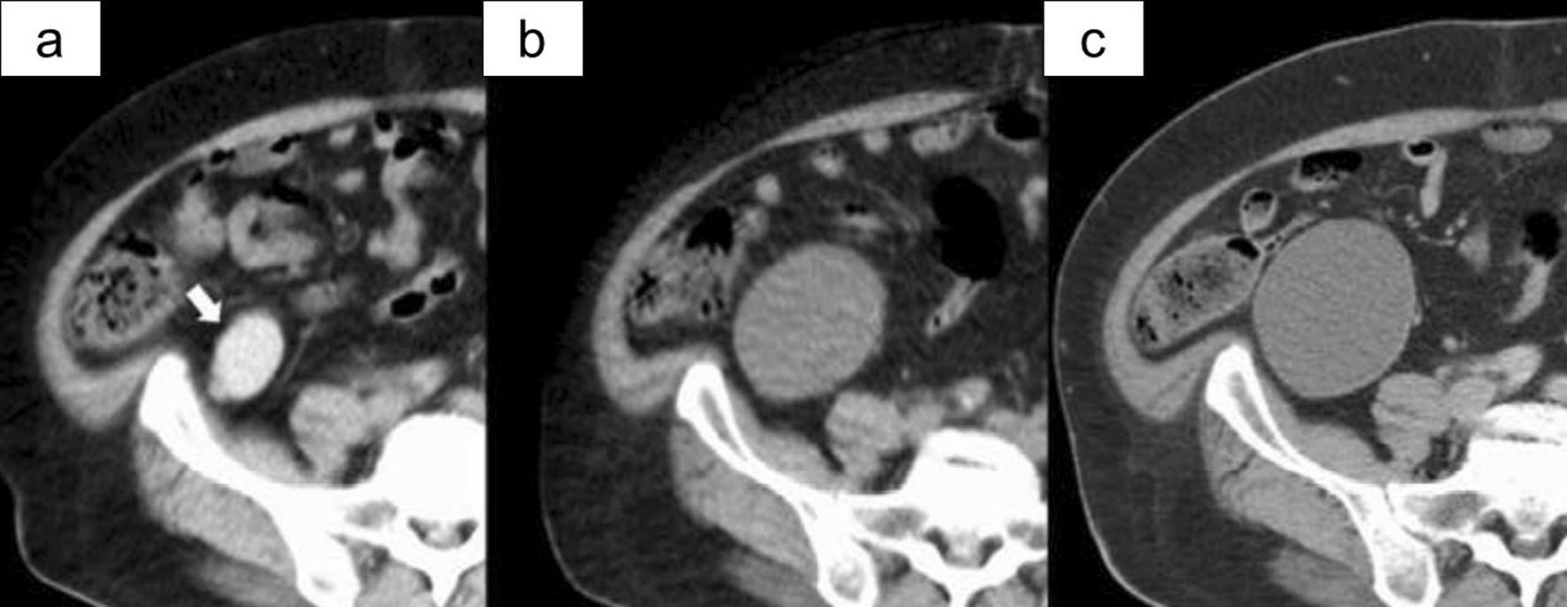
Computed tomography changed over time. Non-enhanced CT performed 3 years previously showed a 5-cm cystic mass with a uniform high density (arrow) (a), while non-enhanced CT performed 6 months previously showed a cystic mass had grown to 8 cm with a uniform low density (b). On the most recent CT scans, the concentration in the cyst was slightly reduced in comparison to these images (CT value: 20.3HU), and the diameter of the cyst had grown to 10 cm (c)
